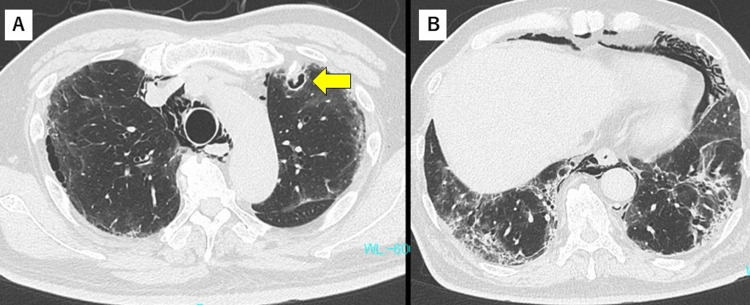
Chest CT findings on day 19 (A) Cavity seen with a small nodule in the left upper lobe (arrow). (B) Pneumomediastinum and fibrosis in lower lungs.
